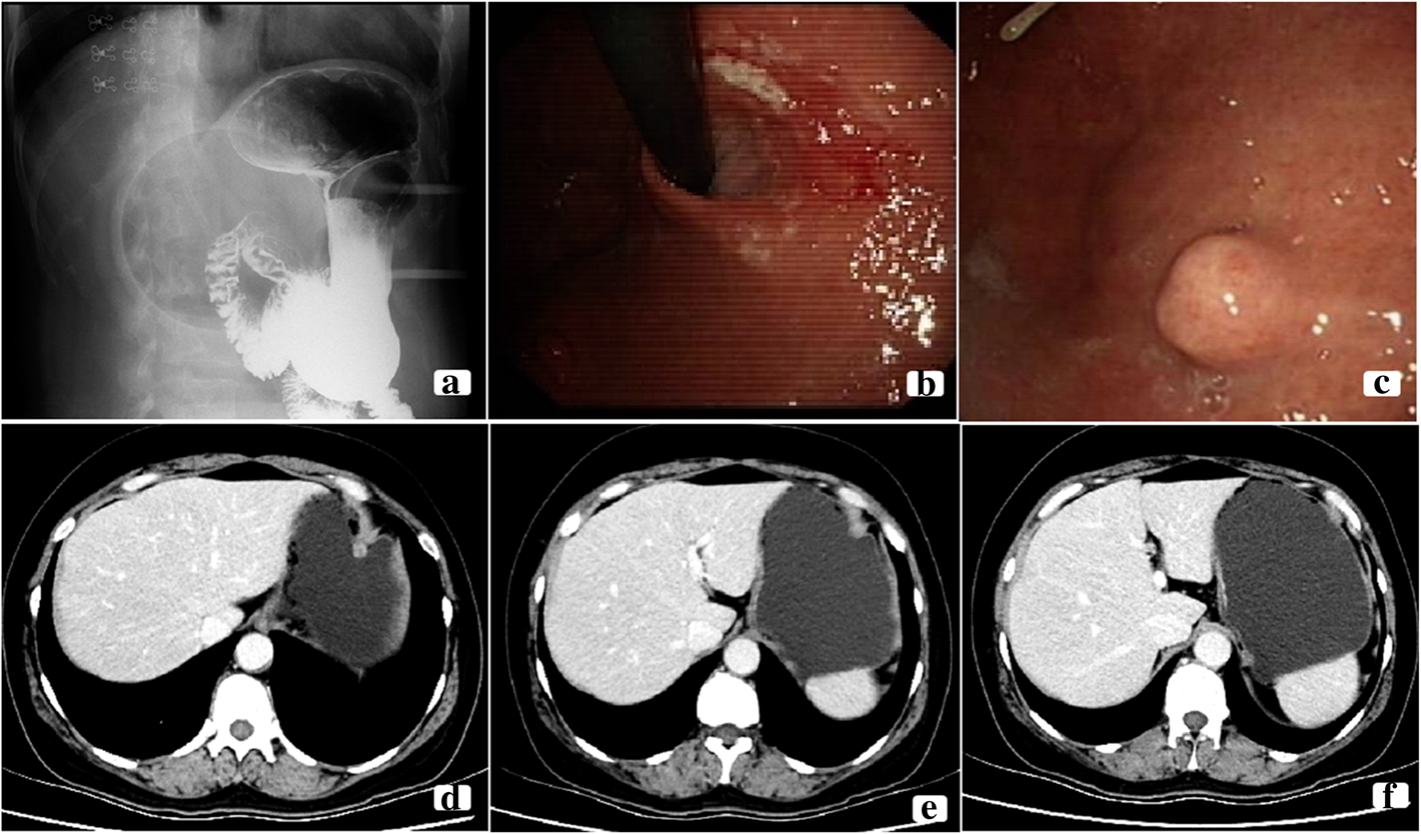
Imaging and endoscopic finds. a Barium swallow showing a small filling defect in the fundus of stomach. b Endoscopic examination showing a linear ulcer in the subcardiac region. c Endoscopic examination showing a polyp in the fundus of stomach. d, e, and f Enhanced abdominal CT scan showing obvious strengthening of the mucosal surface by thickening of the lateral wall of the lesser curvature of the fundus and body of stomach
